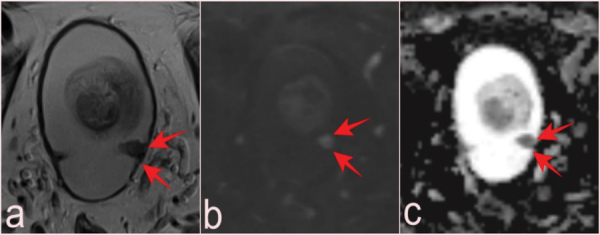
T2-WI (a), DWI (b), and ADC map (c) of a lesion with low-grade pTa tumor pathology reported as VI-RADS 1, which can be missed at the level of mucosal folds in a 69-year-old patient with prostate mpMRI after PSA elevation (arrows). ADC, apparent diffusion coefficient.
